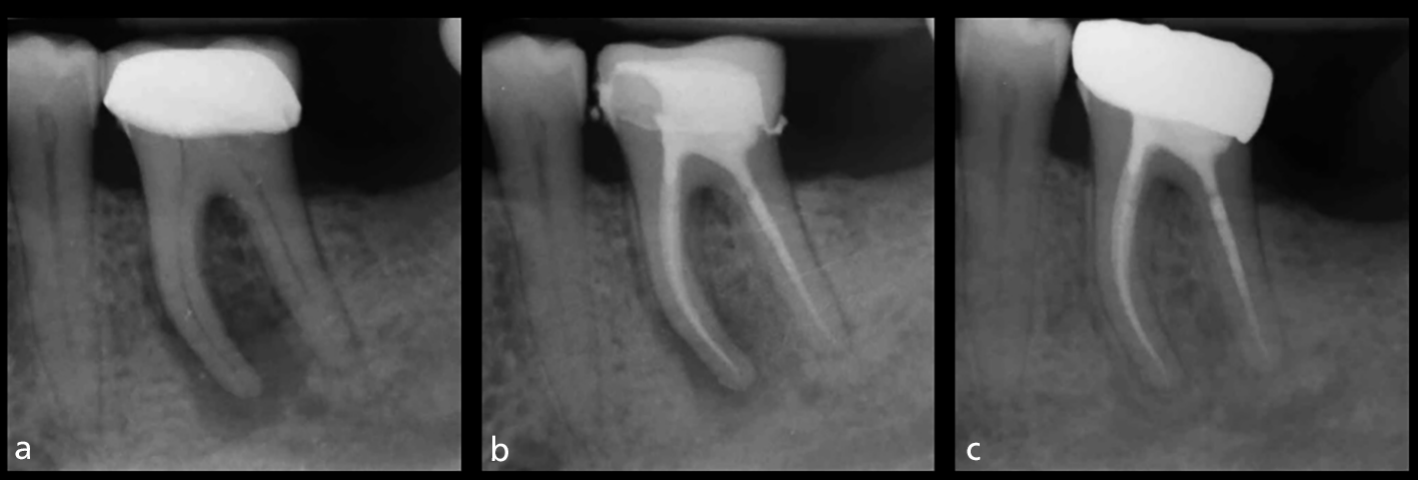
a, b) Primary root canal treatment of this mandibular left first molar was carried out following crown removal and restorability assessment. A new crown was placed following the completion of root canal treatment. c) At the one-year follow-up, there has been complete clinical and radiographic healing, indicating a successful outcome
